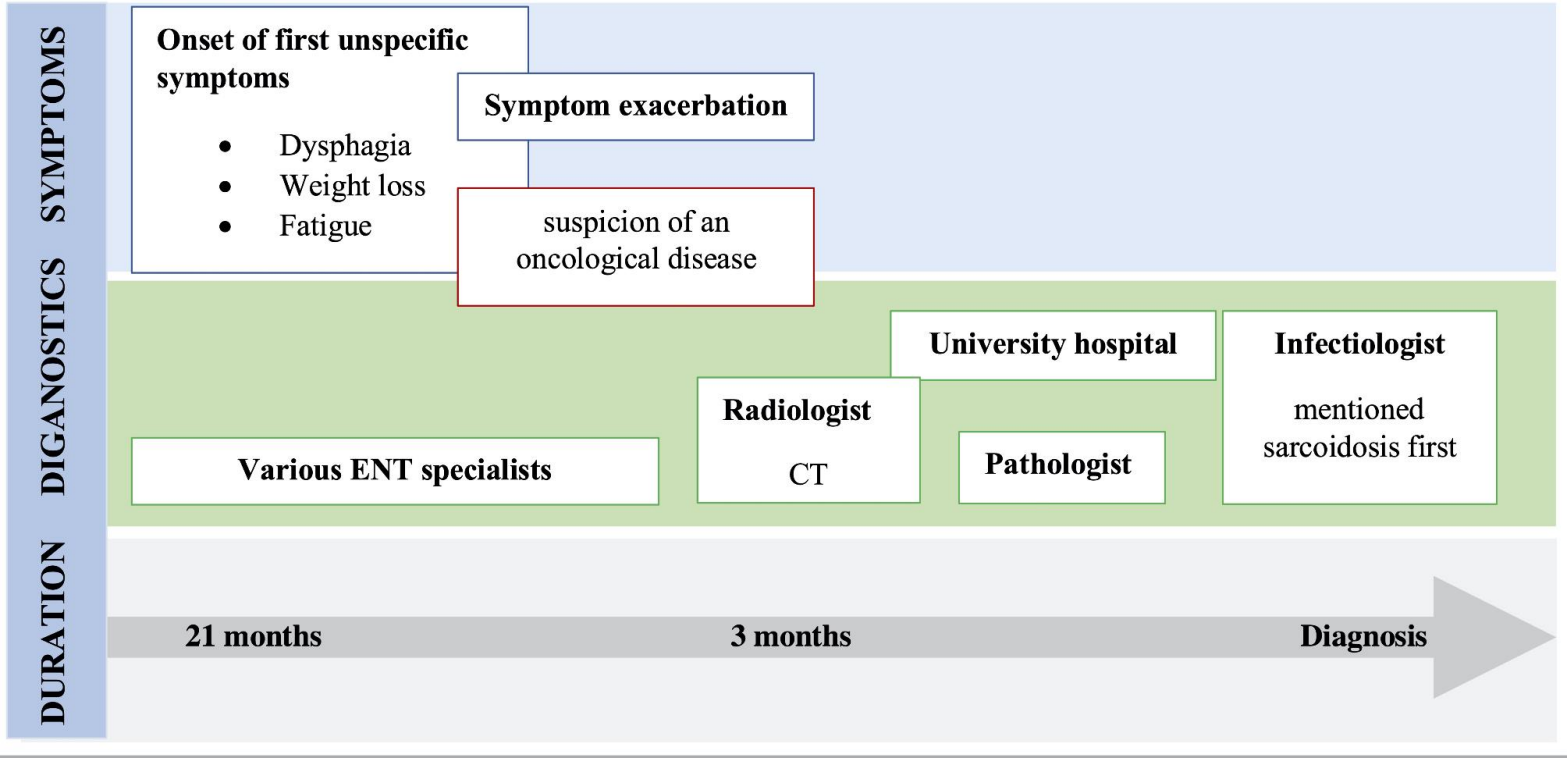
Course over time from first symptoms until diagnosis using one patient’s journey as an example. ENT; ear, nose, and throat, CT; computed tomography
